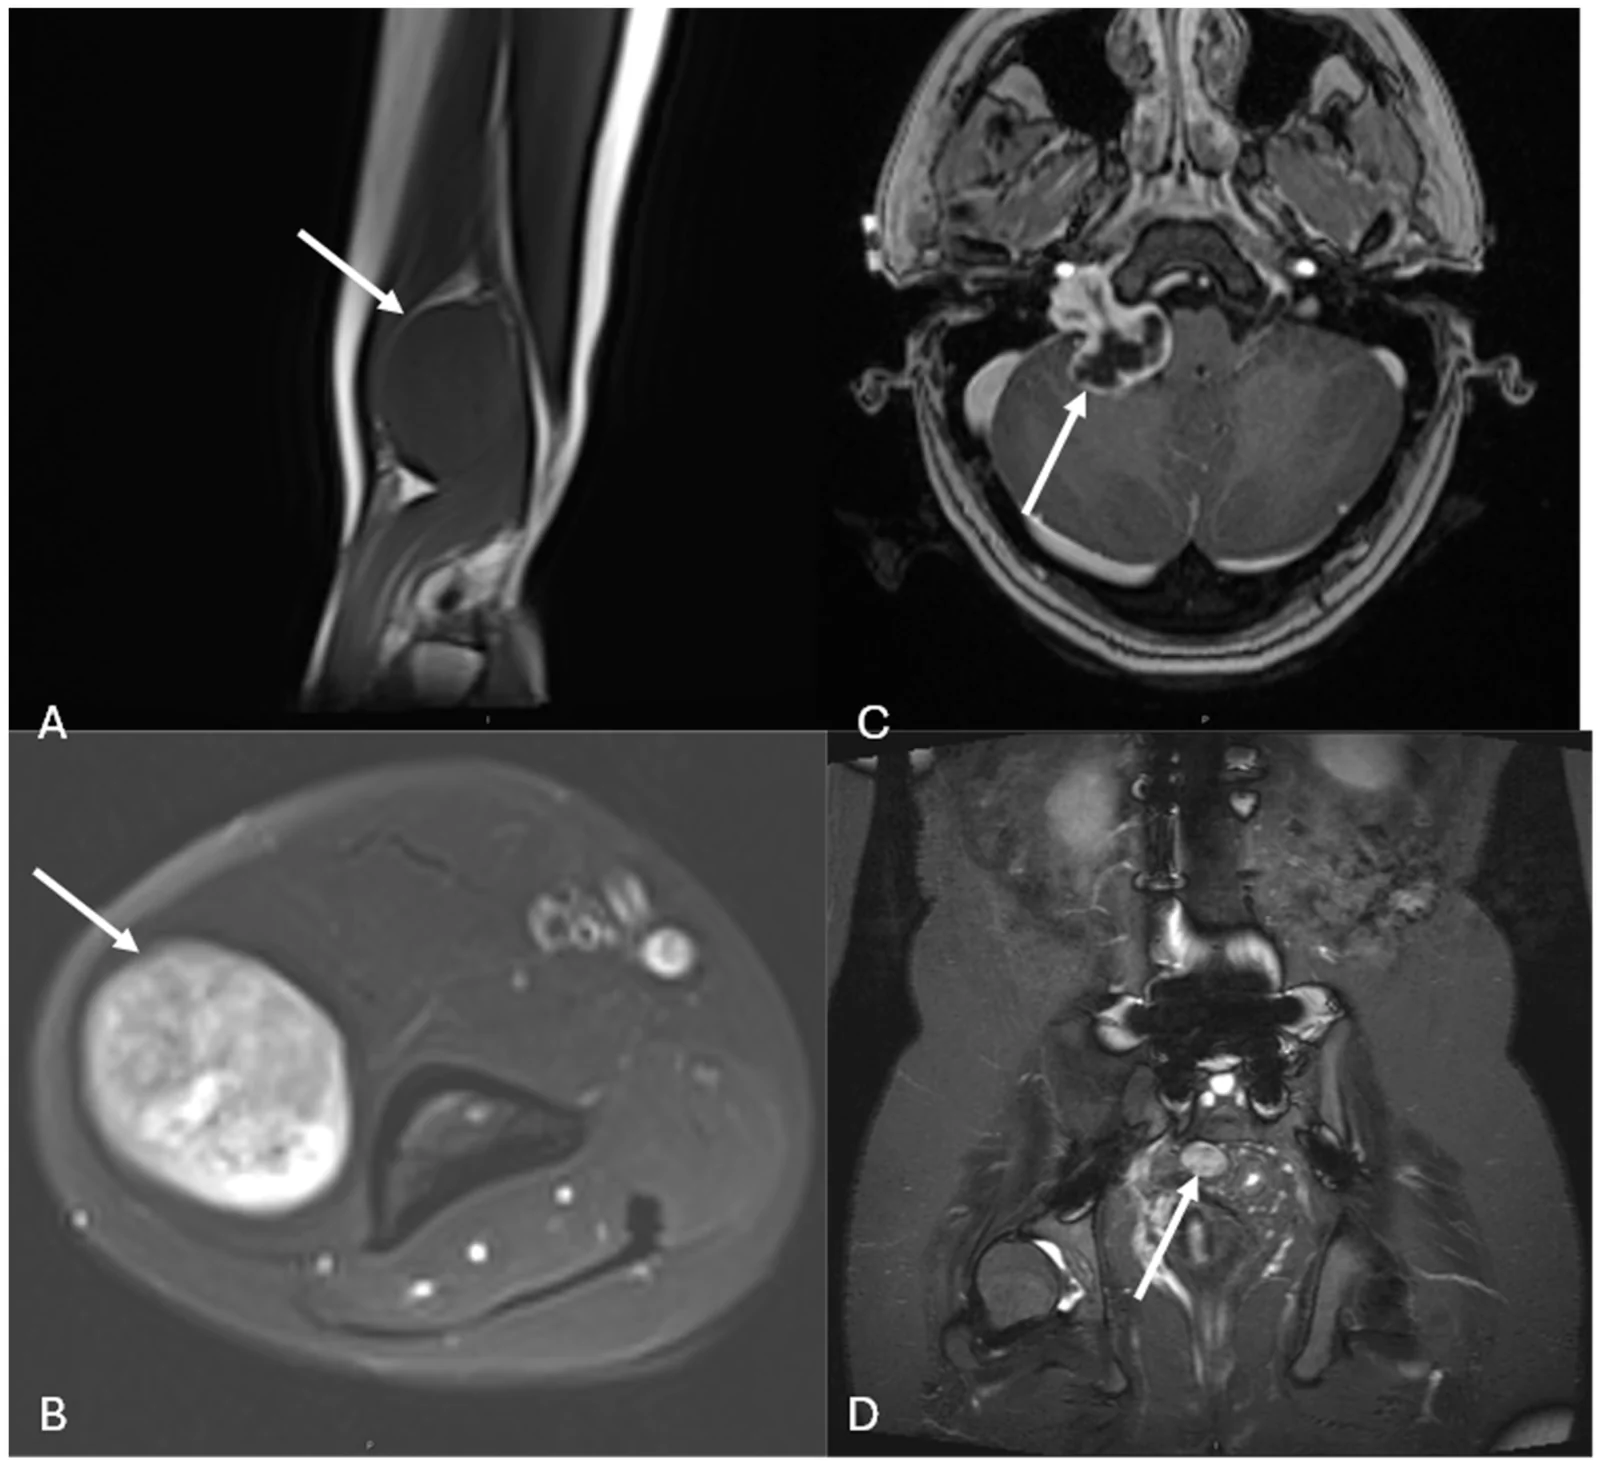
24-year-old female with pathologically proven right distal arm radial nerve schwannoma (arrow on (A,B)), right vagus nerve schwannoma (arrow on (C)) and a midline presacral presumed schwannoma on whole-body MRI (arrow on (D)). No VSs were detected on MRI of the brain and IACs. Genetic testing was not available. As such, the patient was diagnosed with SWN-NOS and managed operatively with resection of the radial nerve schwannoma and debulking of the vagus nerve schwannoma.
